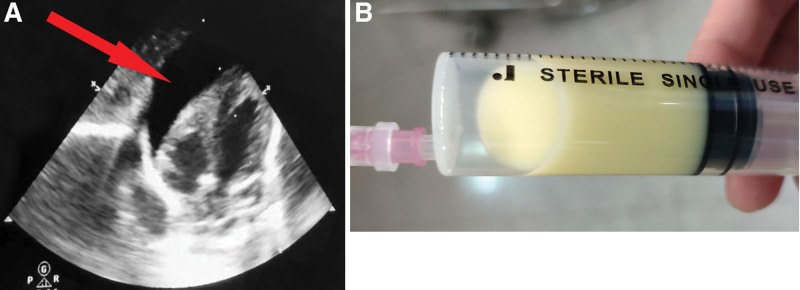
Echocardiogram and appearance of pericardial effusion in case 2. (A) An area of viscous and turbid fluid detected by echocardiography (red arrow); (B) Pericardial effusion appeared chylomicrons.
